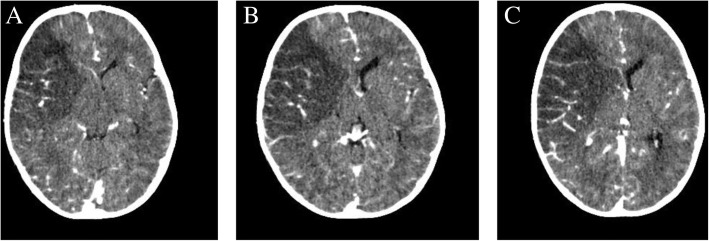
a–c: Computed tomography of the brain showing a cerebral and basal ganglia hypodensity with loss of gray white matter differentiation in the M1 segment of the right middle cerebral artery territory (acute ischemic infarct) and effacement of the right lateral ventricle with a leftwards midline shift
